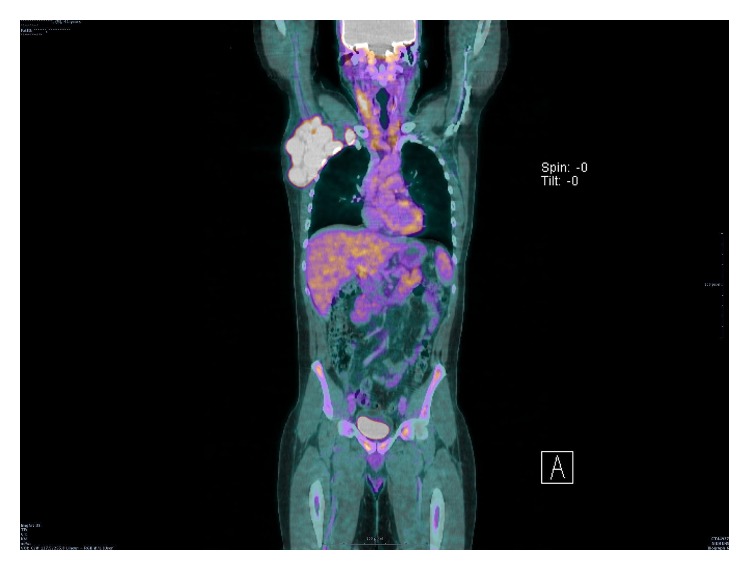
PET CT before treatment. Stage II bulky Hodgkin lymphoma.
